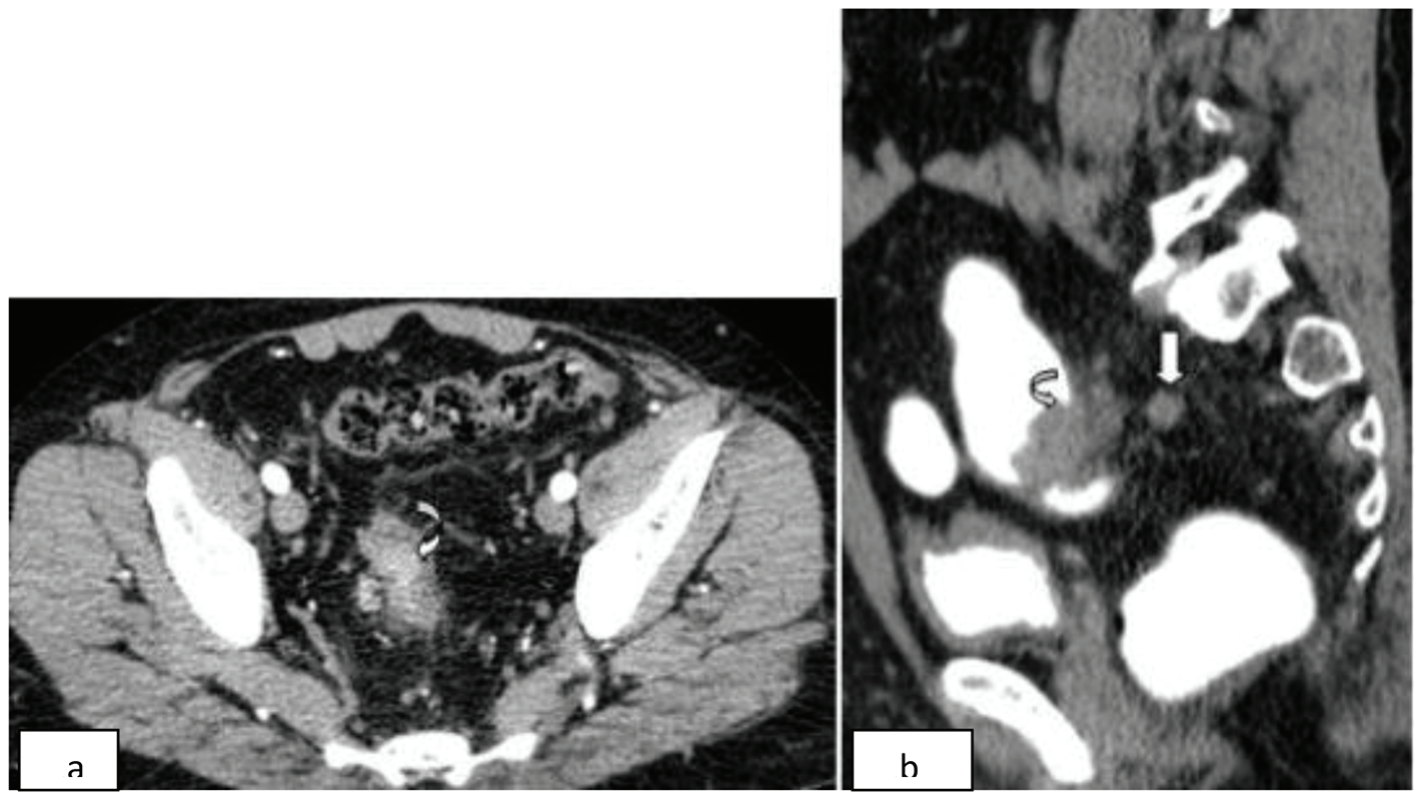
Rectosigmoid mass. a) Contrast-enhanced axial CT in arterial phase shows a well-defined moderately enhancing mass involving the rectosigmoid region (curved arrow). b) Sagittal reformatted CT after rectal contrast shows the polypoidal mass (curved arrow) infiltrating the perirectal fat with an adjacent perirectal lymphadenopathy (straight arrow).
